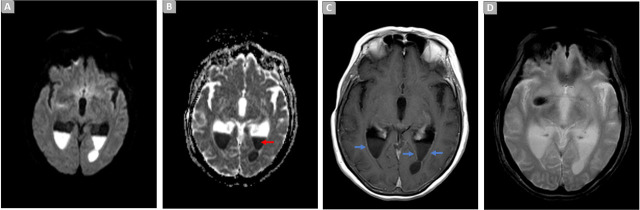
Brain MRI. Axial diffusion weighted image (A), ADC map (B), T1 SE post-contrast (C), and T2*-GRE (D), demonstrating signs of ventriculitis manifested by an increase in dimensions and ‘tension’ of the walls in the occipital and temporal horns of the ventricular system [C, blue arrows]. Content with restricted diffusion and a liquid level in the occipital horns: indicating purulent content [A–B: red arrow]. There is no significant blooming in T2* suggestive of hemorrhage [D].
